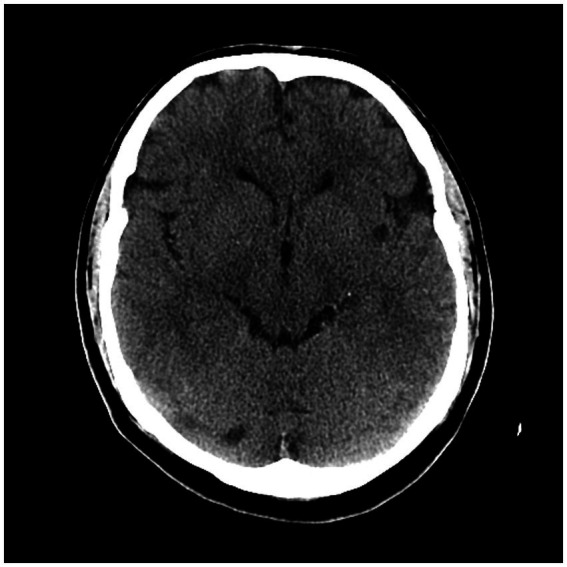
CT scan showing left posterior insular cortex (PIC) ischemic lesion.
